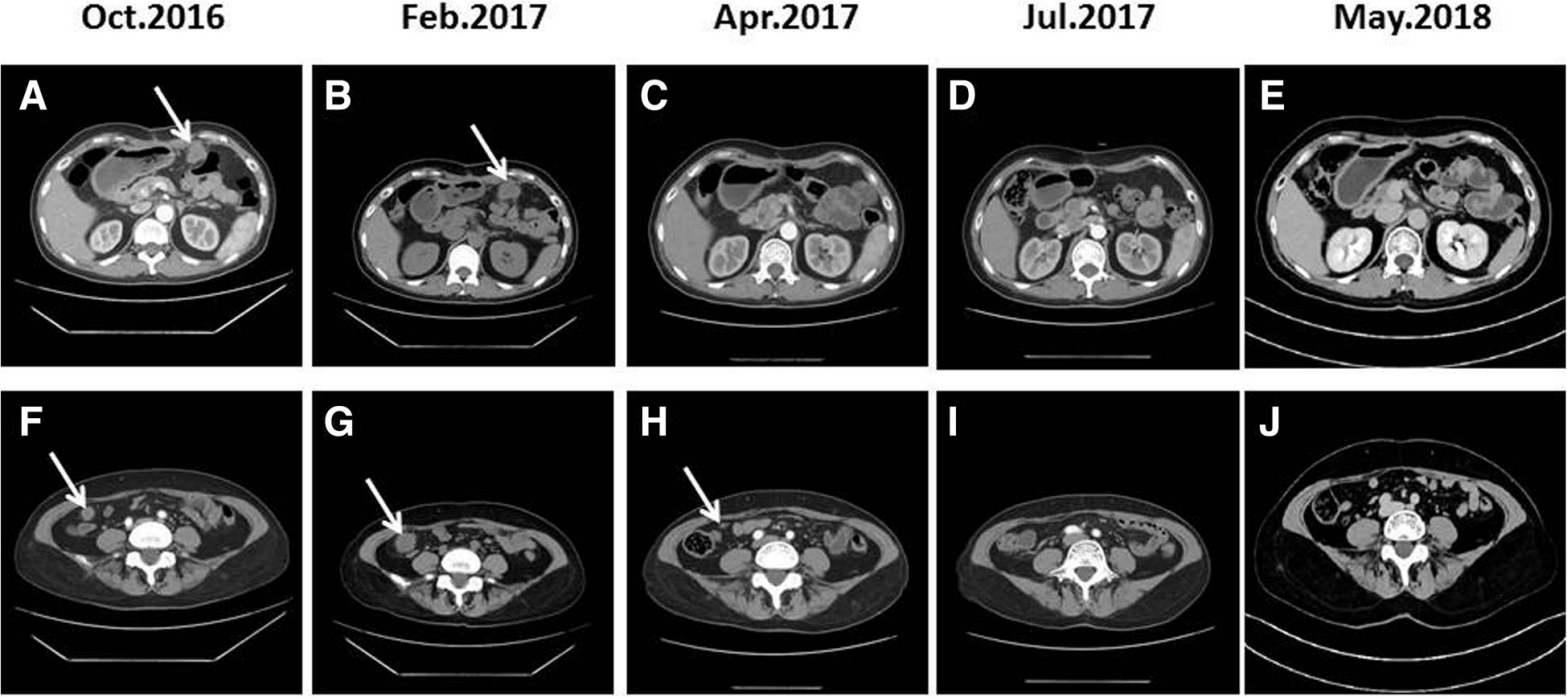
Computed tomography scan shows the different stages of the metastatic lesions in the coelom over time (white arrows). a-e indicate the lesion located in the upper left omentum majus and f-j indicate the lesion located in the lower right peritoneum
